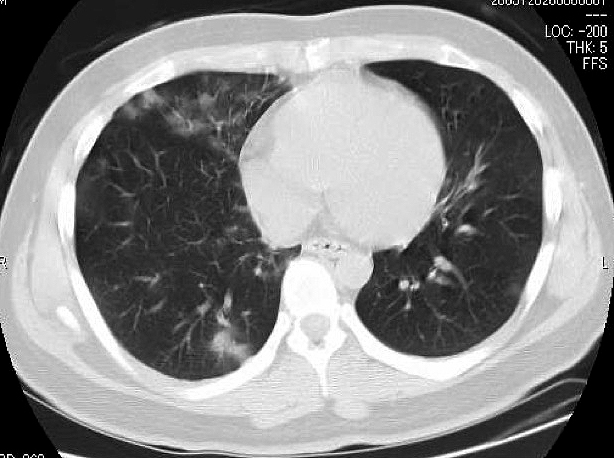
Chest CT on admission, indicating bilateral peripheral multiple ground glass infiltration.
